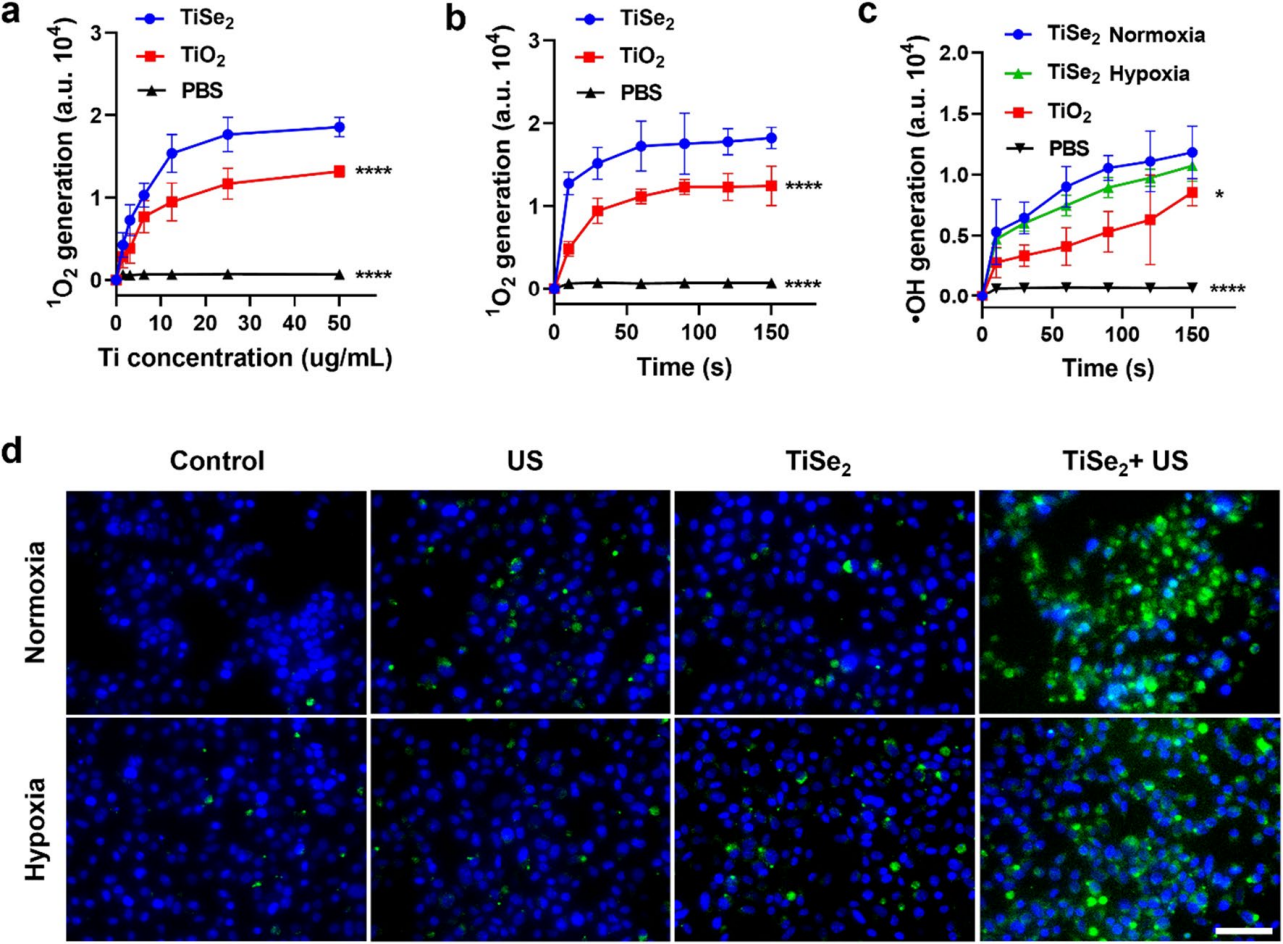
Analyses of in vitro ROS production. Cells were monitored for the dose-dependent (a) and time-dependent generation of ^1^O_2_ (b), as well as the time-dependent generation of ·OH generation (c), and the intracellular production of ROS within Panc02 cells as detected with DCFH-DA (d). US, ultrasound. Scale bar: 50 μm. Data are means ± SD (n = 3), and were compared via one-way ANOVAs with Dunnett’s post hoc test. P* < 0.05, *P* < 0.0001
